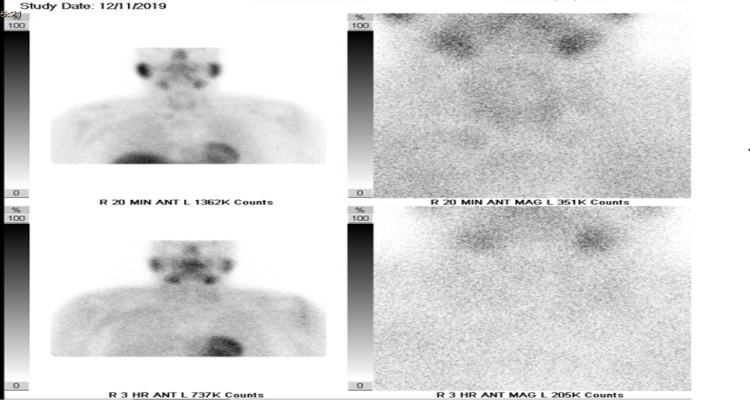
Tc-99m Sestamibi Impression: No evidence of parathyroid adenoma. There is focal area of increased sestamibi uptake in the posterior portion of left lung lower lobe. No findings on CT images (lung window) at this location. This finding likely represents a radioactive sestamibi embolus during IV injection of sestamibi.
